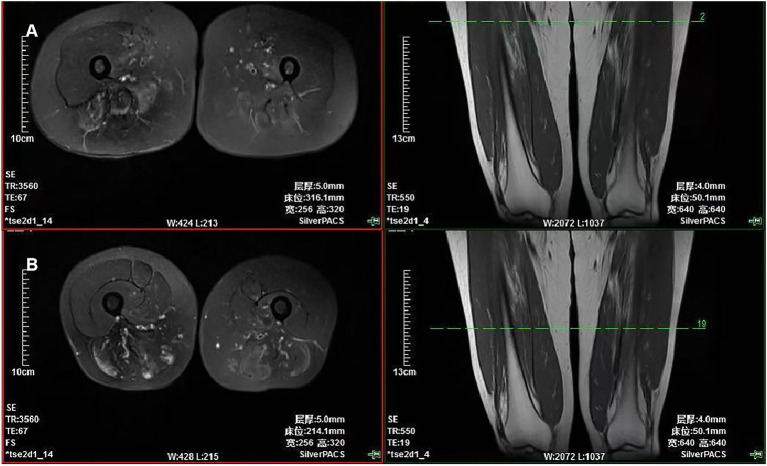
MRI of the patient’s bilateral femoral muscles: (A) muscular atrophy; (B) muscular edema.
